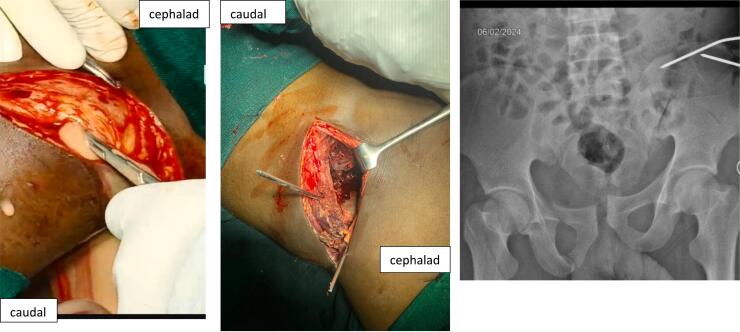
Left: Image captured after making the incision and opening the fascia over the external oblique muscle. Middle: Image taken after surgical debridement and k-wire fixation. Right: AP pelvic radiograph following temporary k-wire fixation.
